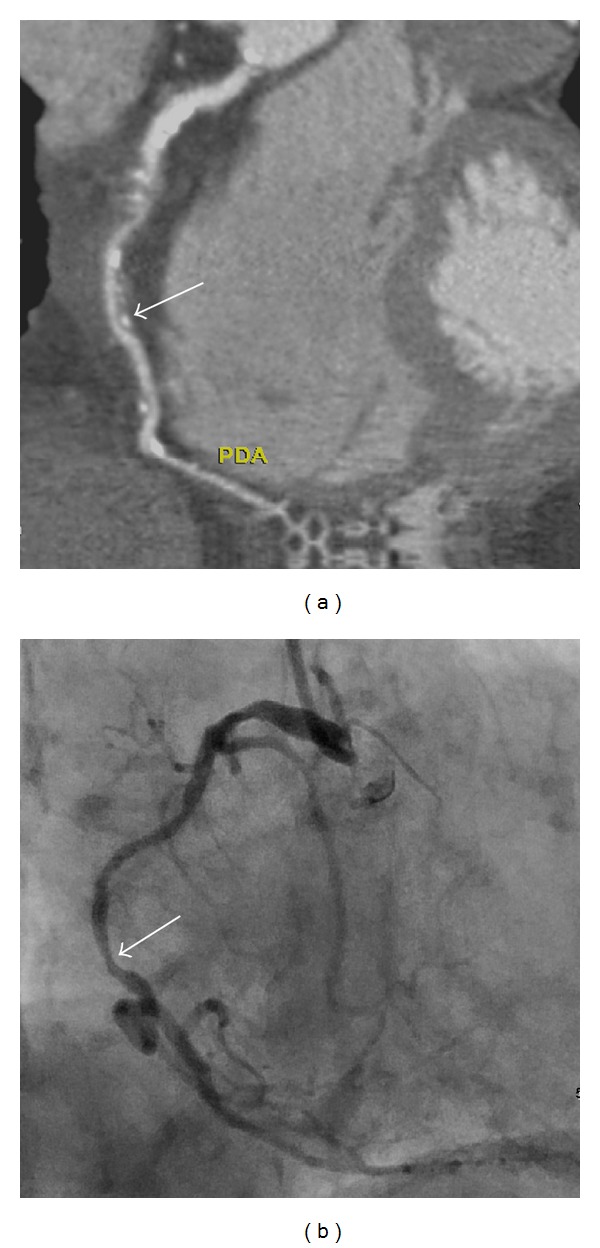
A 86-year-old female with two-vessel coronary artery disease, total calcium score was 1278. The calcium score was 325 over the right coronary artery (RCA). (a) A computed tomographic angiogram shows mixed plaques over the middle third of RCA with 54% stenosis (white arrow). (b) Conventional coronary arteriogram confirms the moderate stenosis over the proximal as well as middle (arrow) third of RCA.
